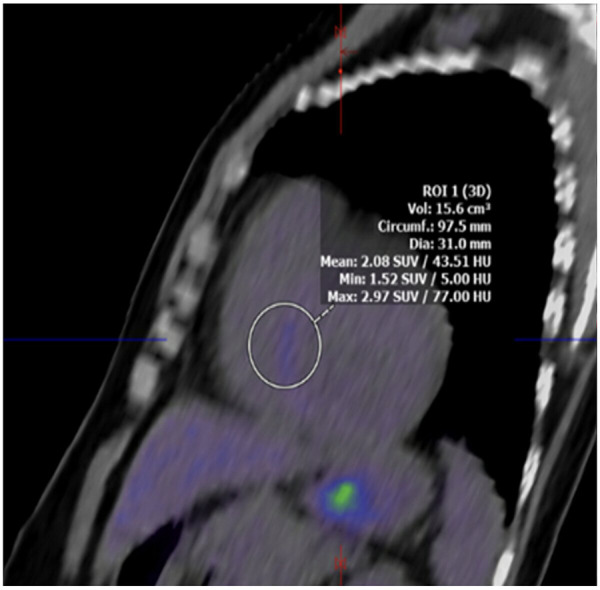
Positron emission tomography (PET) in a patient with arrhythmogenic mitral valve prolapse. The figure shows focal myocardial 18F-fluorodeoxyglucose (FDG) uptake, consistent with metabolically active myocardial involvement. The image shows a region of interest (ROI) within the basal inferolateral left ventricular wall with a volume of 15.6 cm^3^, circumference of 97.5 mm, and a diameter of 31.0 mm. Measured uptake values within the ROI include a mean standardized uptake value (SUV) of 2.08, minimum SUV of 1.52, and a maximum SUV of 2.97, supporting localized inflammatory activity in the myocardial tissue adjacent to the mitral valve apparatus. PET, positron emission tomography; FDG, fluorodeoxyglucose; ROI, region of interest.
